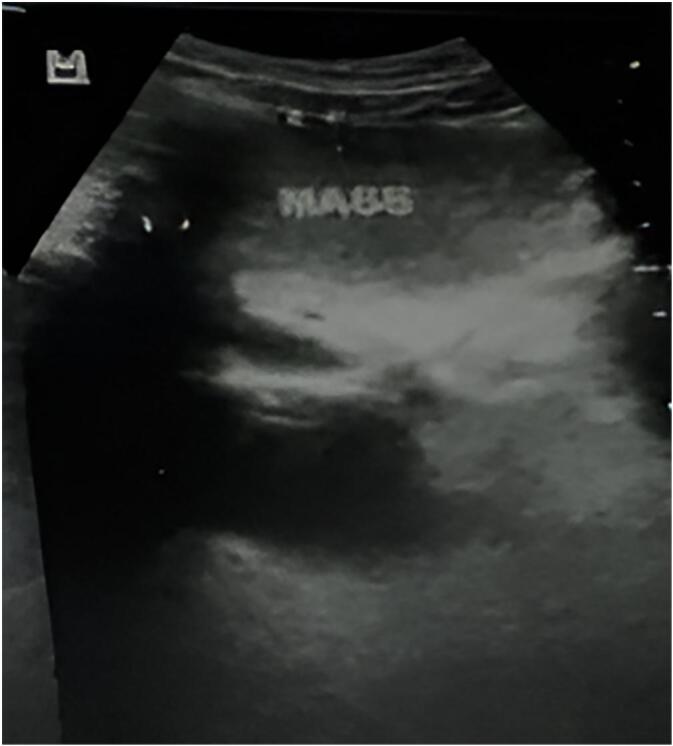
Representative sonographic image showing a well-defined, homogeneously hyperechoic intra-abdominal mass, consistent with a lipoma.
